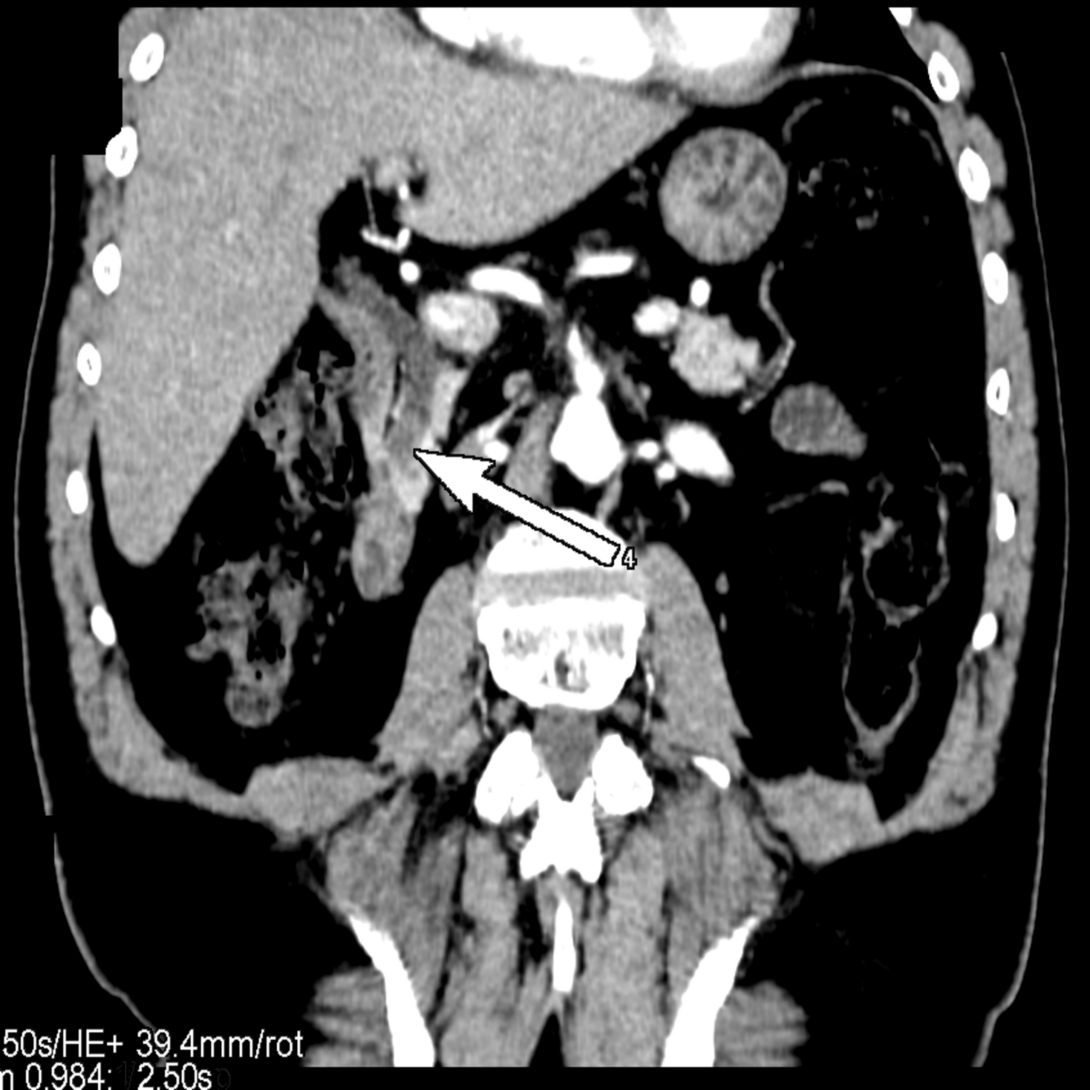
Computed tomography, coronary view; dilated extrahepatic bile duct (white arrow).
